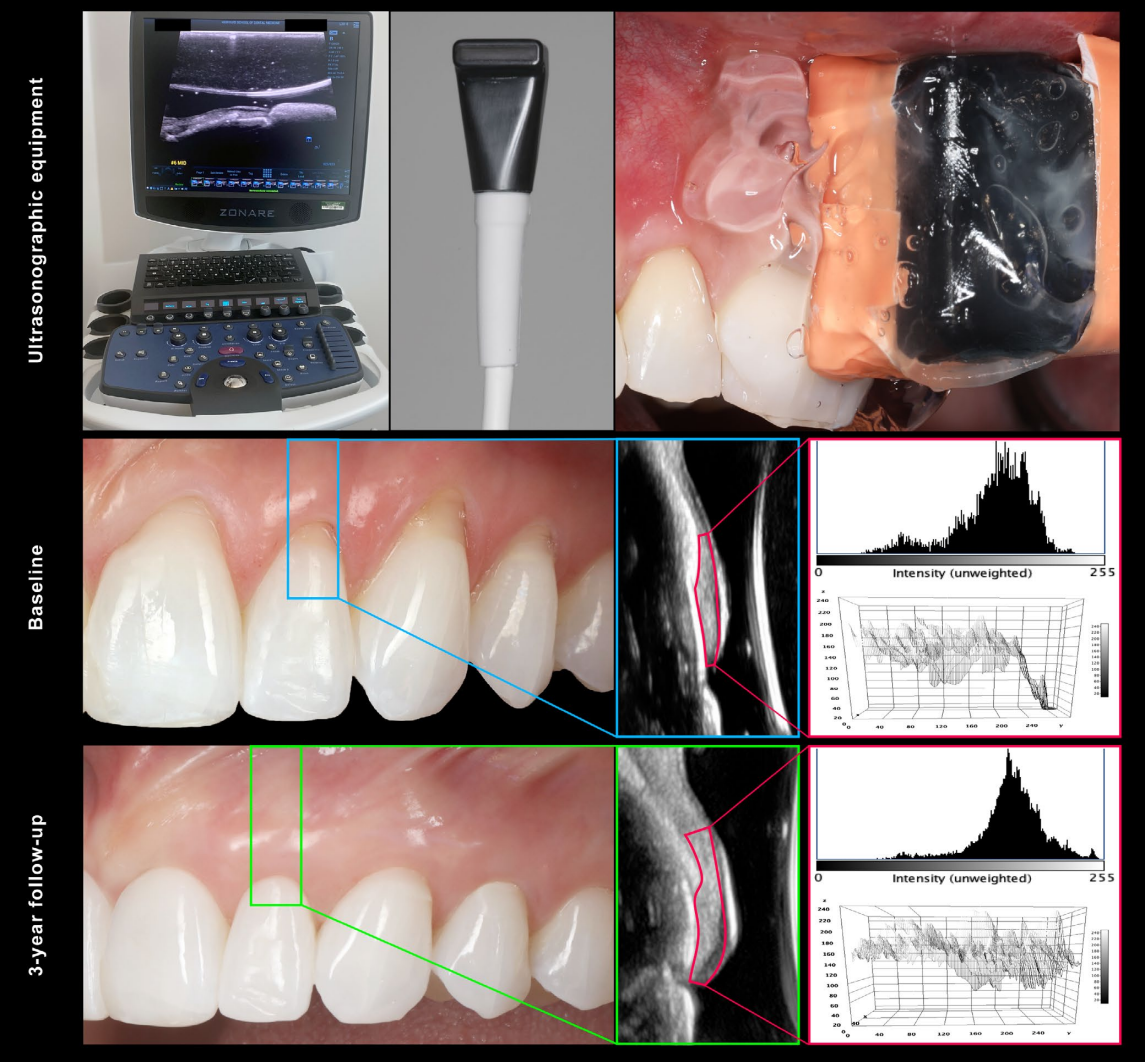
Ultrasonographic grey‐scale analysis at the regions of interest. In this example, it is possible to appreciate that the mean echo intensity (EI) of the coronal soft tissue of the lateral incisor prior to the intervention was 127.2 GL, while at the 3‐year follow‐up the respective EI was 164.4 GL, indicating a substantial change within the structure and density of the augmented soft tissue. Adapted with permission from John Wiley and Sons (Mascardo et al. 2024).
